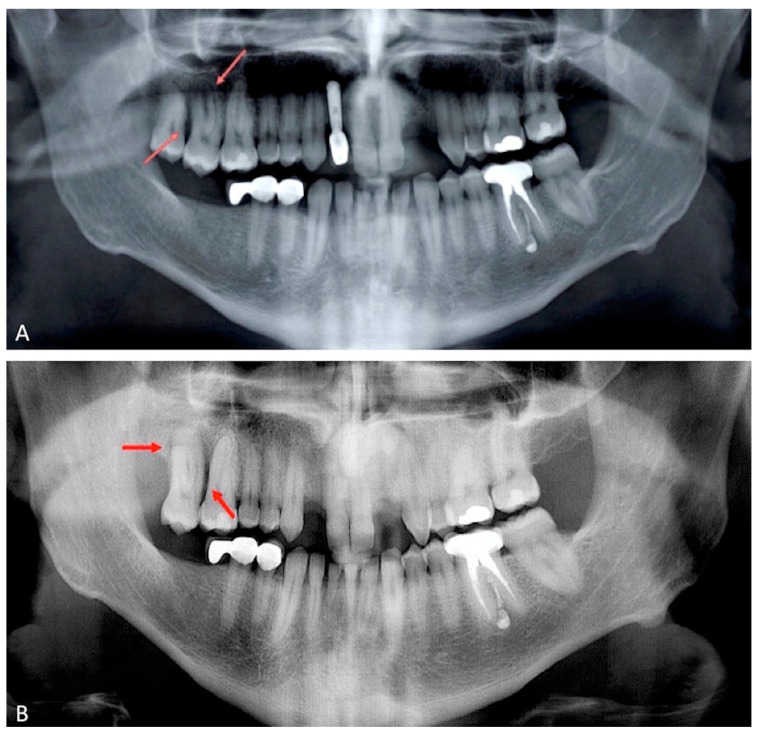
Orthopantomography of 2018; bone defects were not observed between 1.6. and 1.7. (panel A). Orthopantomography repeated in 2021. A severe horizontal bone loss and absence of tooth decay were observed. A horizontal radiolucent line in the third apical root was present (panel B). Red arrows indicate the lesions.
